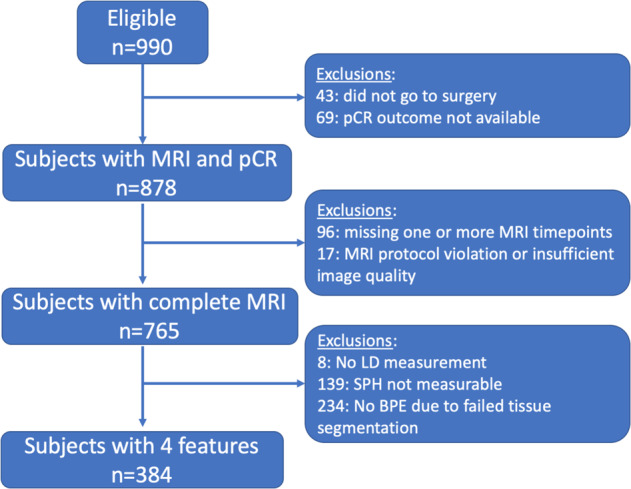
Study subject exclusion criteria. Out of 17 patients excluded for MRI protocol violation or insufficient quality, 10 had protocol violation or technique failure, 6 had obvious motion or were re-positioned after contrast injection, and 1 patient could not tolerate MRI. Image quality issues contributing to the exclusion of BPE values (*n* = 86) were insufficient fat suppression (*n* = 47) or coil inhomogeneity artifact (brightness on the outer edge of the breast, *n* = 37), or both (*n* = 2). The remaining number of exclusions (*n* = 148) were due to the segmentation failure. pCR pathologic complete response, LD longest diameter, SPH sphericity, BPE background parenchymal enhancement.
